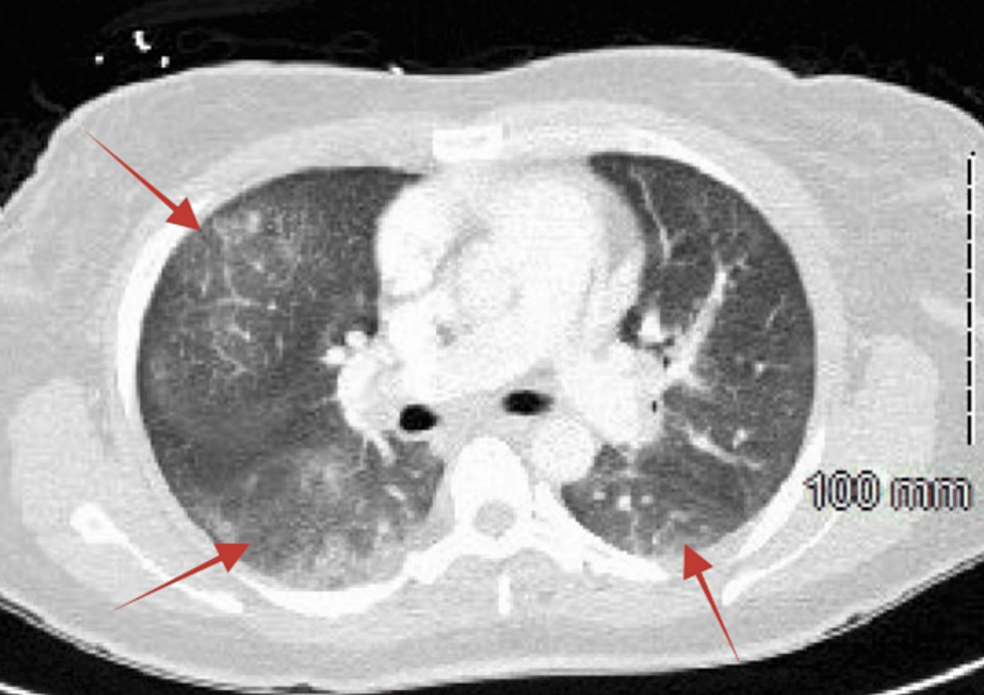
The chest CT shows multifocal bilateral patchy opacities (arrows), predominantly involving the lower lobes and right middle lobe.
